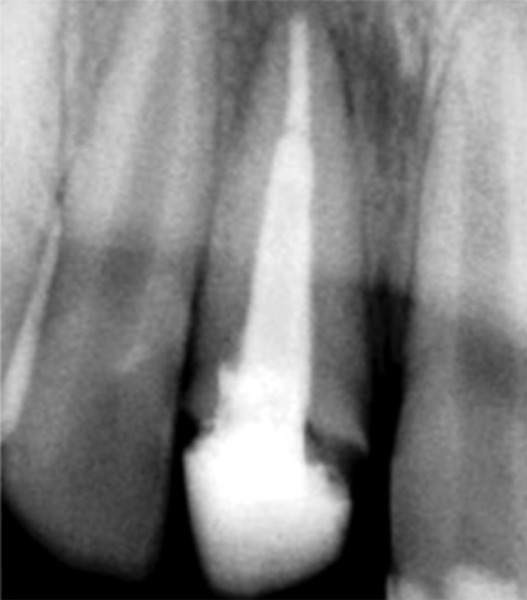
Radiographic confirmation during core build up
